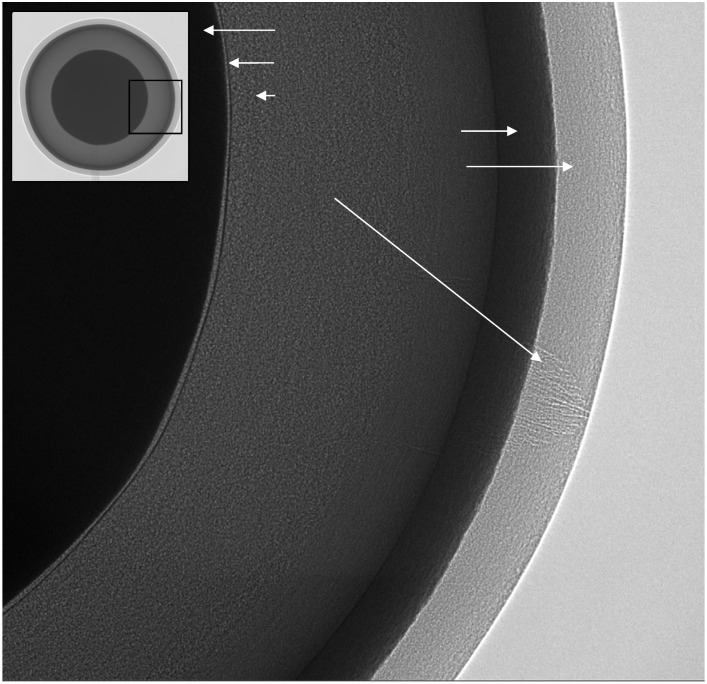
2D Phase-contrast X-ray image of a fuel pellet consisting of layers of pyrolitic carbon and silicon carbide on a zirconia core. In the main image a crack can be seen, highlighted by phase-contrast, in the outer pyrolitic carbon layer. The inset is a lower magnification image providing an overview of the whole sample, approximately 800 μm in diameter.
